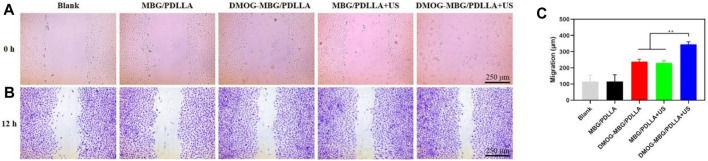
The effects of the scaffolds and ultrasound stimulation on the migration of HUVECs after cultured for 12 h, (A) 0 hours (B) 12 hours and (C) quantitative analysis of migration.
